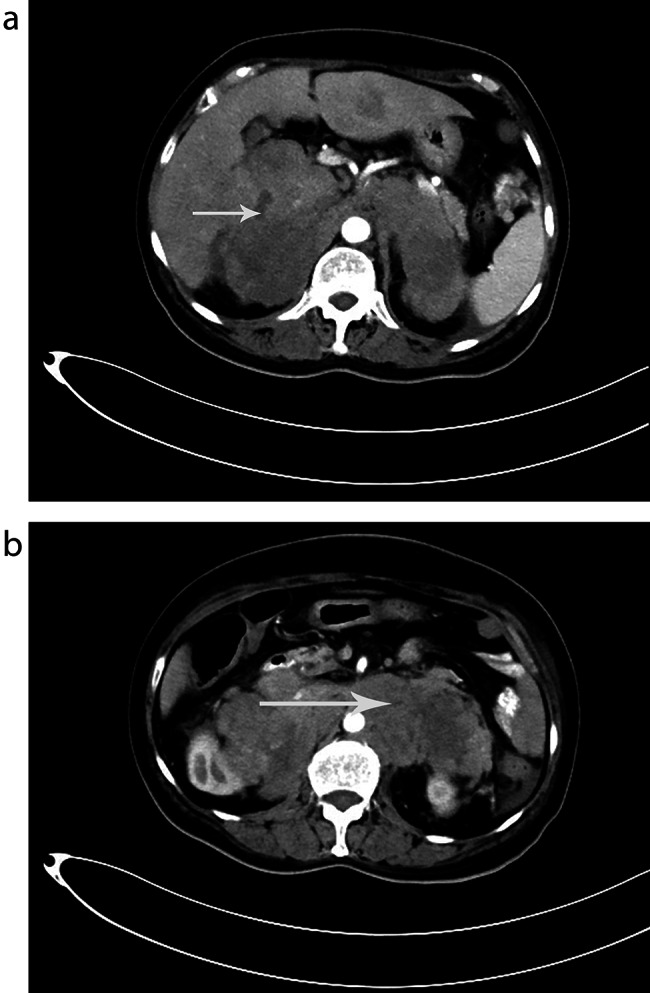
CT revealed liver(A,arrow) and retroperitoneal lymph node metastases(B,arrow)
